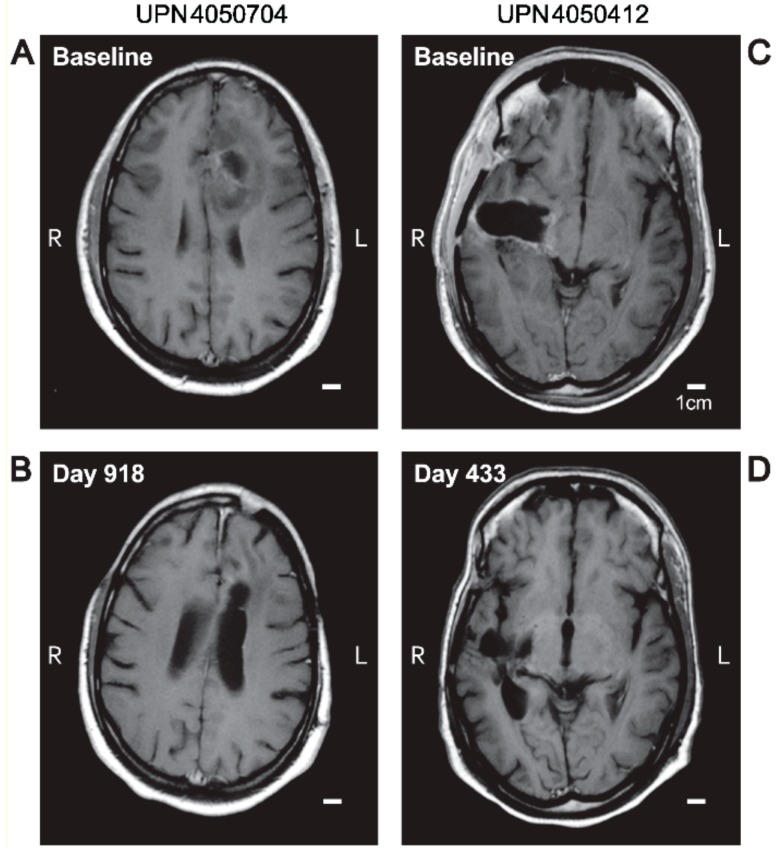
MRI-response of target lesion in OT101 treated *R/R* high-grade glioma patients. Depicted are T1-weighted spin echo (SE) post-contrast axial MRI images at baseline vs. post-treatment with OT101 at 433 days post randomization to their respective OT101 dose cohorts. Panels A and B: Unique patient number (UPN) 405-0704 (anaplastic astrocytoma (AA), WHO Grade III) achieved a CR. Panels C and D: UPN4050412 (glioblastoma multiforme (GBM), WHO Grade IV) achieved a PR.
